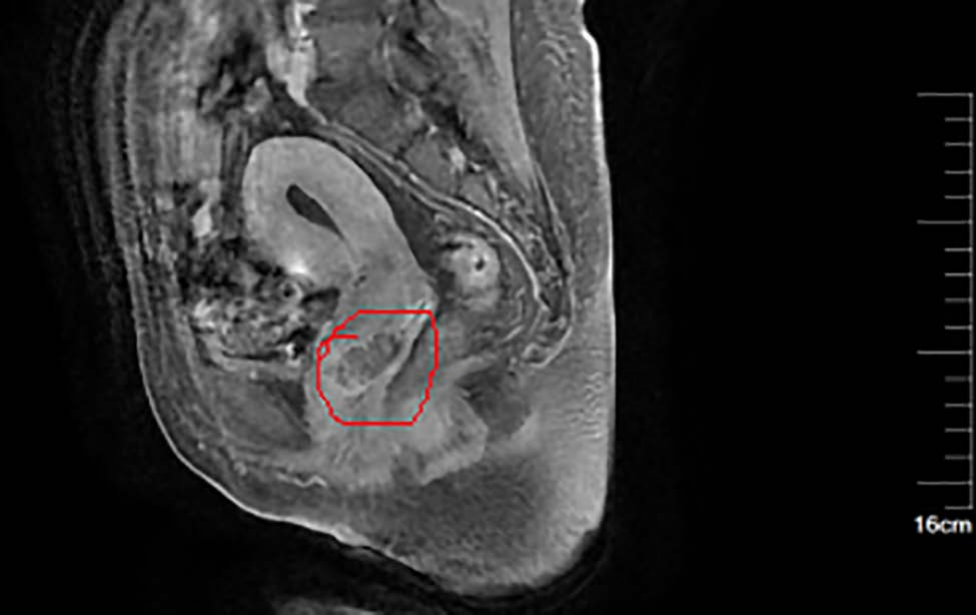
Pelvic MRI showed that there was a high possibility of vaginal malignancy invading the cervix, and abnormal signal shadows in the left adnexal area suggest a high possibility of metastasis.
